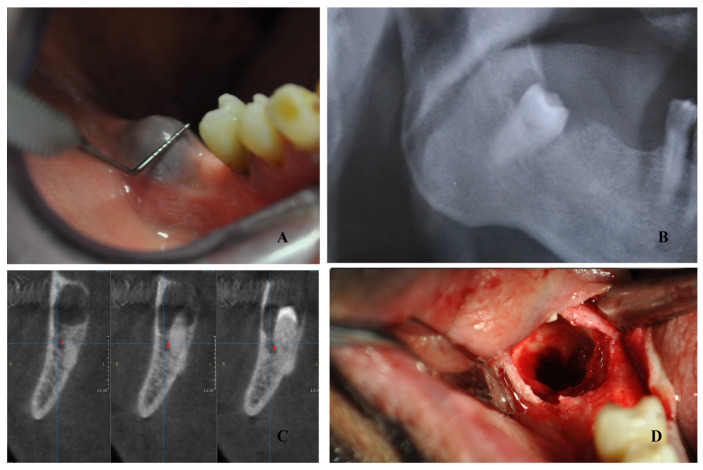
A rounded swelling area in the right mandibular third molar site (A). Panoramic radiograph image with impacted mandibular right third molar surrounded by a radiolucent lesion (B). CBCT reveals alveolar nerve involvement (C). Intraoperative image after cyst and third molar removal (D).
